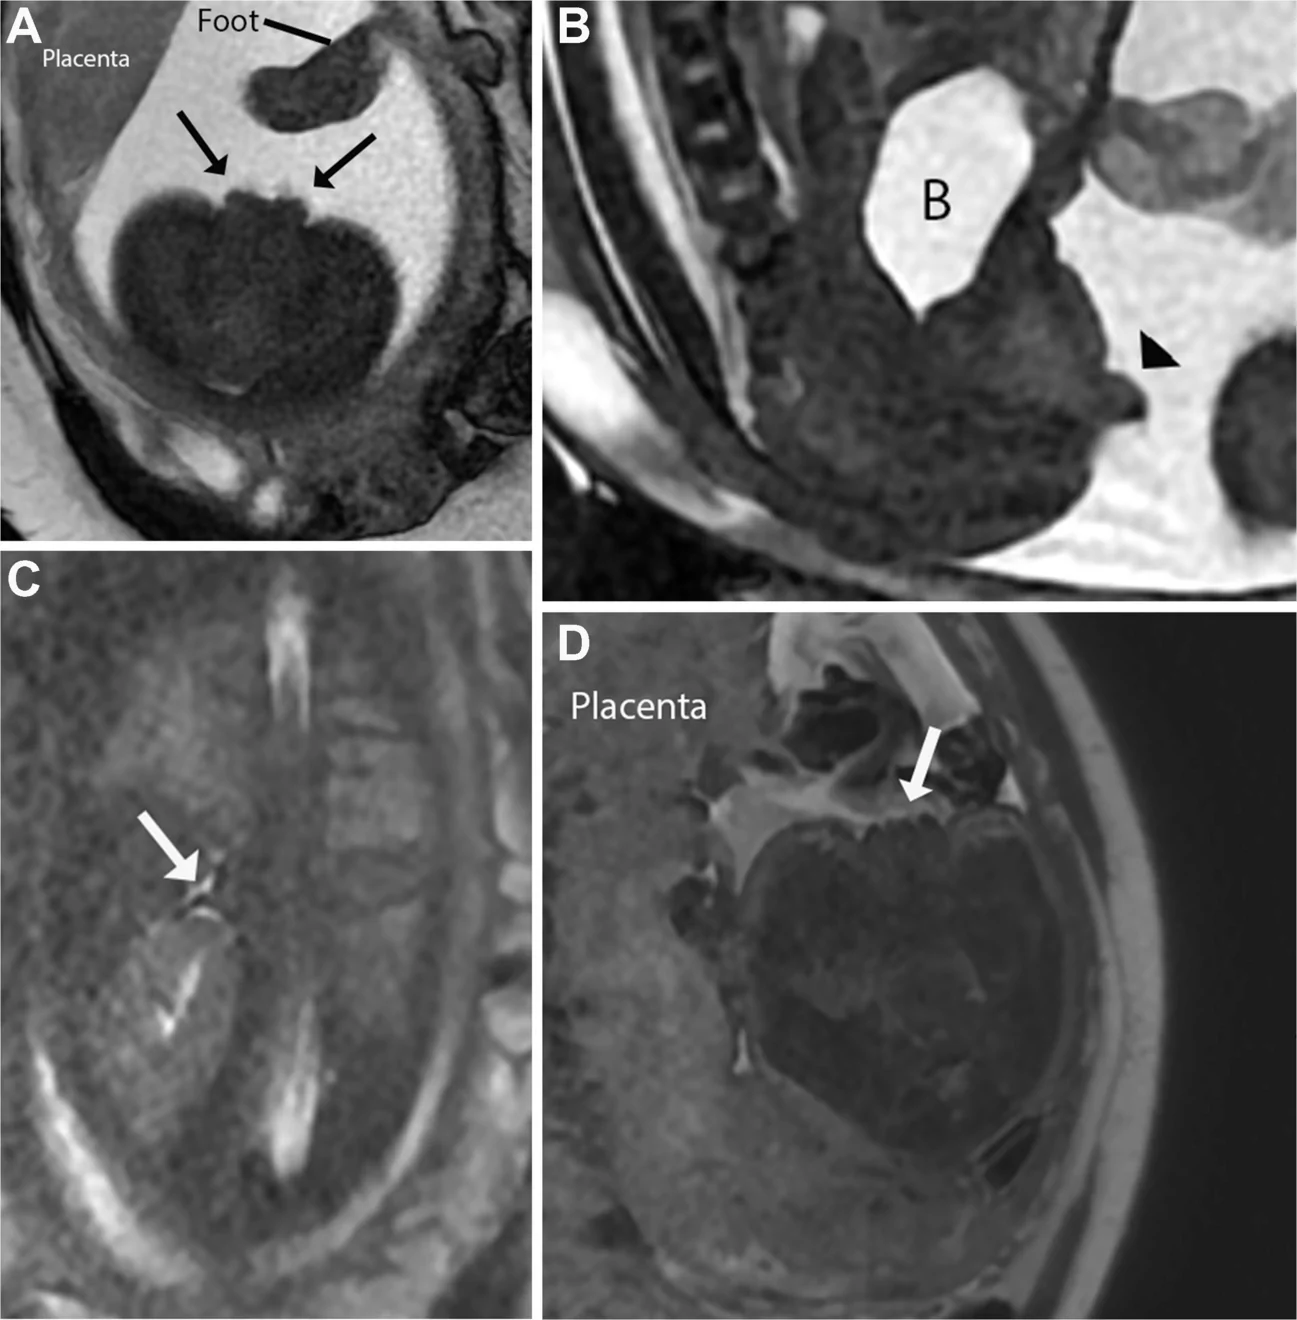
Atypical virilized genitalia identified by fetal MRI in a 46,XX fetus at 24 weeks gestation. Axial (a) and sagittal (b) balanced free precession T2-weighted images show labia majora (*black arrows*, a) and clitoromegaly (*arrowhead*, b). *B*, urinary bladder. c Coronal T2-weighted single-shot fast-spin echo of right adrenal gland appears normal (*white arrow*). No abnormality in the left suprarenal space (*not shown*) or elsewhere. Primary considerations include congenital adrenal hyperplasia or maternal sources for hyperandrogenism. d For comparison, normal appearance of fetal genitalia in a different 25-week GA 46,XX fetus. Axial T2-weighted SSFSE through the level of the perineum shows normal labia majora and minora (*white arrow*), without phallic structure
